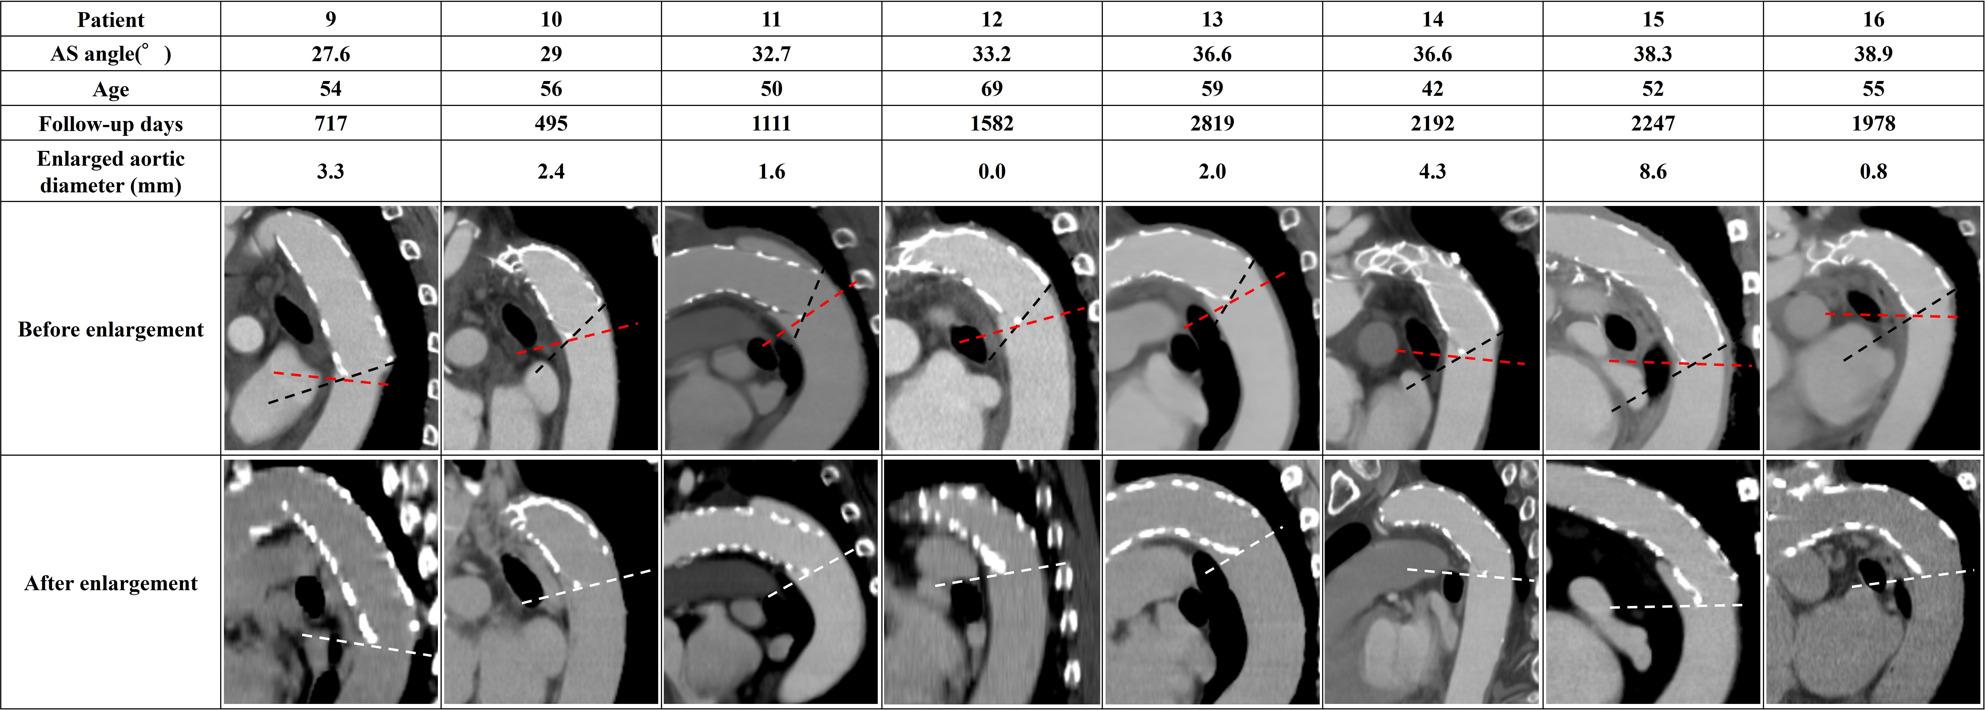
Patient-specific AS angles and CT views (Patients 9–16)
